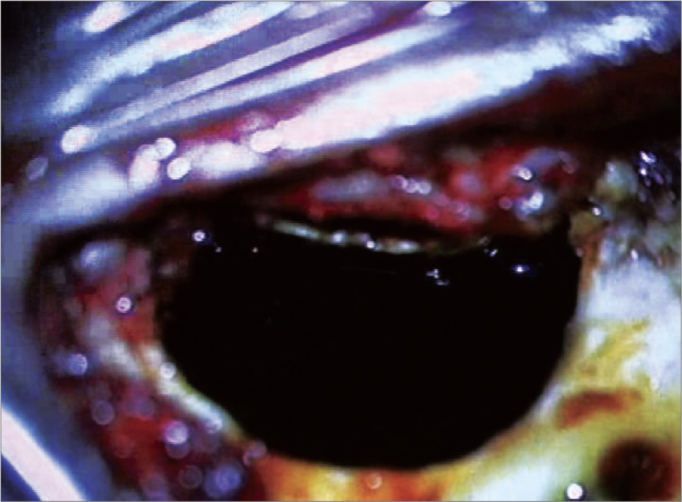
Ilustration of irrigation of the mastoid cavity and middle ear with povidone-iodine solution (10% povidone-iodine / 1% active iodine).10Endovenous administration of dexamethasone (8 mg) before approaching the middle ear via a cochleostomy or through the round window;11Application of topical triamcinolone (40mg/ml) over the round window;12Opening the membrane of the round window; if this approach is impossible, the endosteous is opened by means of a cochleostomy;13Positioning the internal component on its well;14Preparation of the fascia graft; making a pinhole central orifice to allow the electrode to pass snugly to be placed in the cochleostomy/round window site;15Insertion of the electrode slowly and continuously during three minutes through the hole in the graft;16Positioning the muscle graft around the electrode to seal the cochleostomy. Placing bone dust to close the posterior tympanotomy;17Positioning the ground electrode under the muscle-periosteum flap;18Closure with Vicryl^™^ 3.0 sutures on the Palva flap planes and subcutaneous tissue; skin closure with Nylon 4.0;19Cleaning of the patient and placing an external compressive dressing;20Impedance testing, neural response telemetry (NRT), and a transorbital incidence radiograph are done to confirm the position of the intracochlear electrode.
